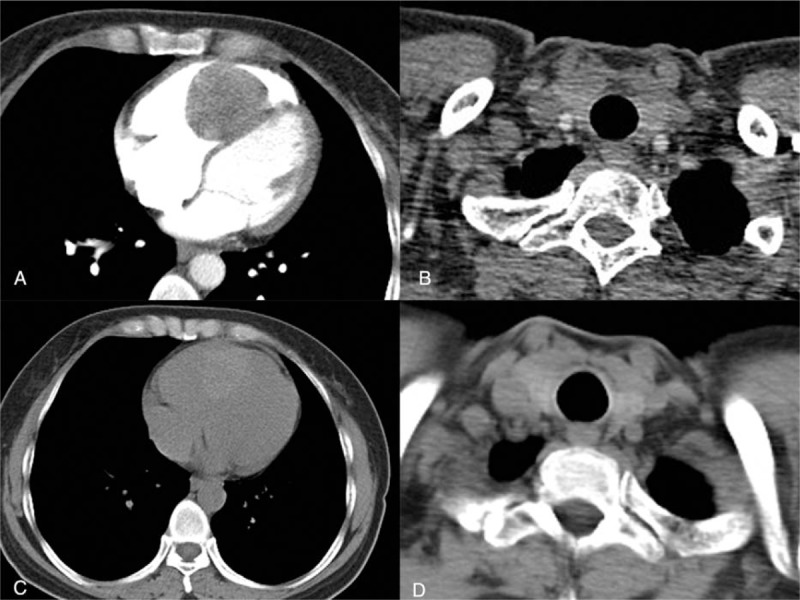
Imaging characteristics of the ectopic thyroid gland in our patient. (A, B) A filling defect is noted in the right ventricle on a contrast-enhanced computed tomography (CT) scan. The mass has a similar enhancement as the cervical thyroid. (C, D) The mass has a similar intensity to the cervical thyroid on the plain CT scan. CT = computed tomography.
